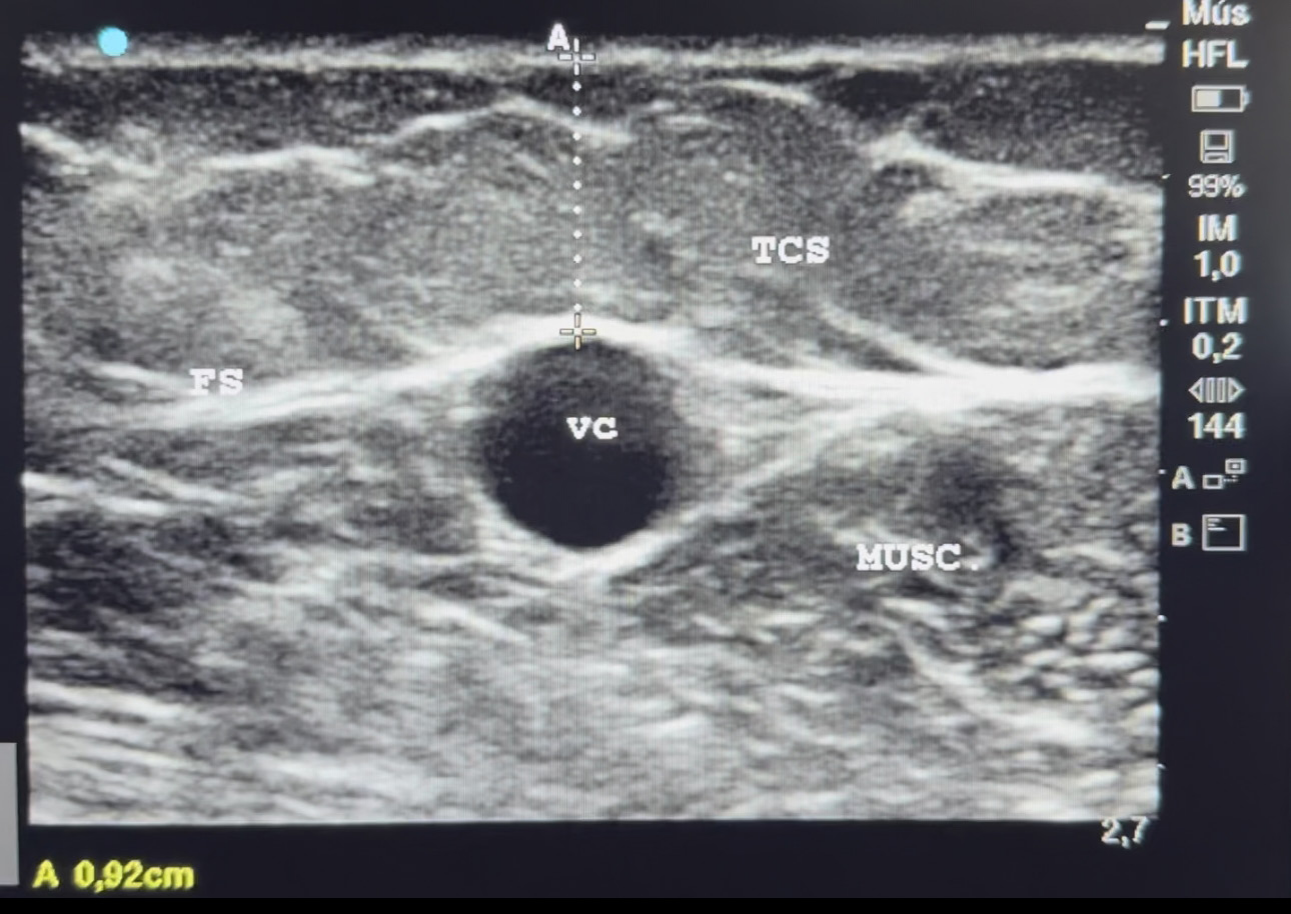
Preoperative ultrasonographic appearance: transverse image including section from skin to the cephalic vein in the arm. The broken line indicates the skin-vein distance exceeding 6 mm. The letters mark examination findings: FS: superficial fascia; TCS: subcutaneous cellular tissues; VC: cephalic vein; MUSC: muscle.
